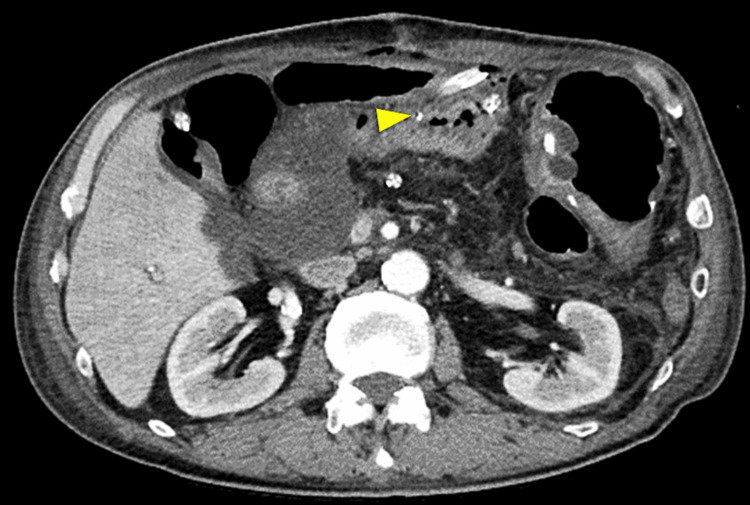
Enhanced abdominal computed tomography of Case 3 performed 5 days after the reoperation. Computed tomography showed the distal end of the pancreatic stent located within the jejunal loop (arrowhead).
